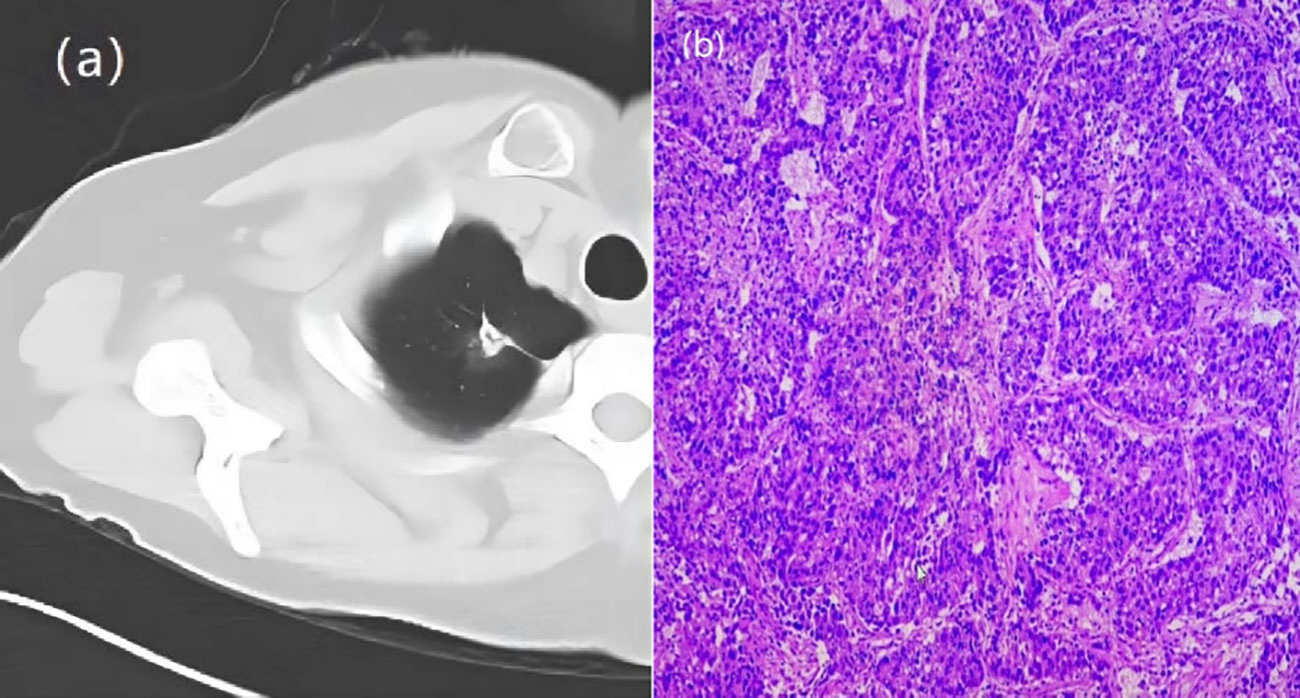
A 66-year-old woman with a GGN. CT showed lobulation, pleural traction, burr. (A) Chest scan. (B) pathologically confirmed for IAC.
